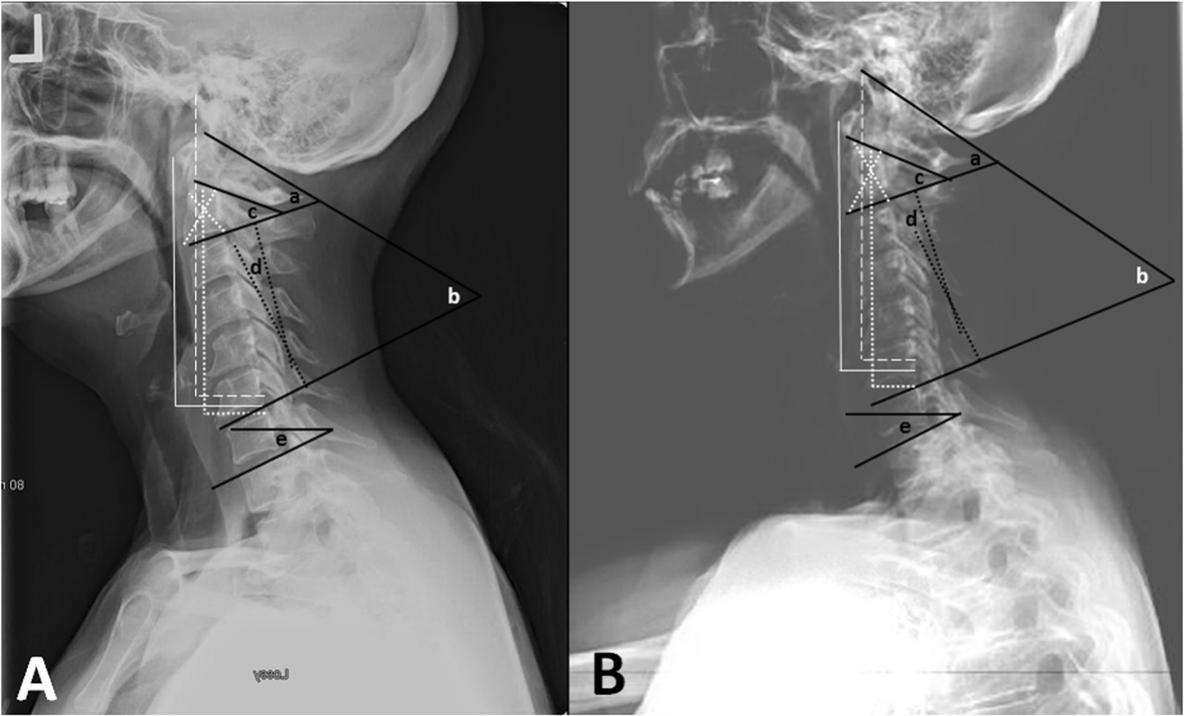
a Cervical lateral radiograph; b Detail of 36-inch lateral radiograph showing measurements (a) Occiput-C2 sagittal Cobb angle, (b) Occiput-C7 sagittal Cobb angle, (c) C1-C2 sagittal Cobb angle, (d) C2-C7 sagittal Cobb angle, (e) T1 tilt. Horizontal solid white line: C1-C7 Sagittal Vertical Axis - distance between plumb line dropped from anterior tubercle of C1 and posterior superior corner of C7; Horizontal white dotted line: C2-C7 Sagittal Vertical Axis—distance between plumb line dropped from centroid of C2 and posterior superior corner of C7; White dashed line: Center of Gravity-C7 Sagittal Vertical Axis—distance between plumb line dropped from anterior margin of external auditory meatus and posterior superior corner of C7
